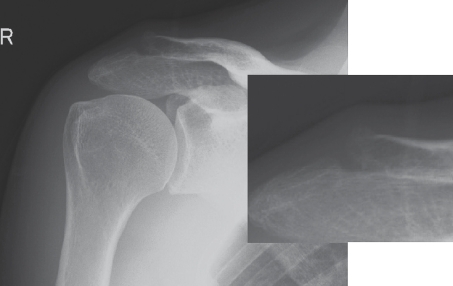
Case presentation. Plain radiographs showing osteosclerosis of the distal end of the clavicle and a cyst in the subchondral bone indicate degenerative arthritis of the acromioclavicular joint.
